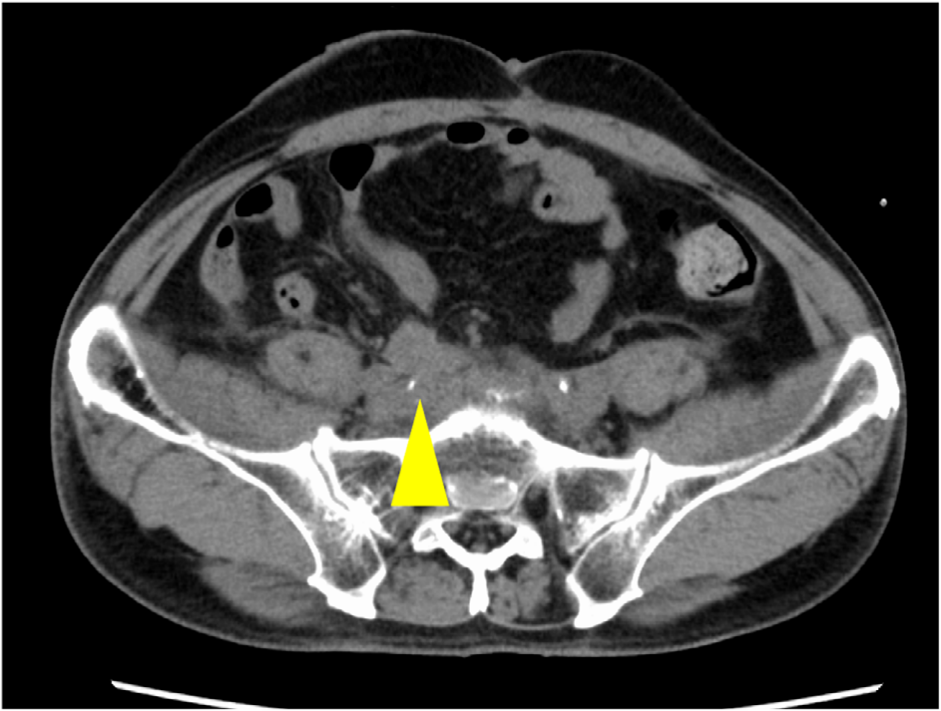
Re‐enlargement of the recurrent mass at the uretero‐ileal anastomosis revealed by axial CT (yellow arrowhead).
